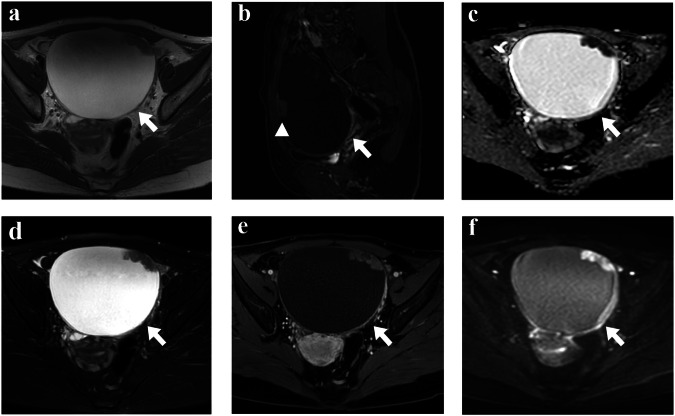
The MR images of a 35-year-old CCC female (FIGO IA stage) with endometriosis were shown as follows. Axial T2WI and axial FS-T2WI (a, d), demonstrated the left ovary had a big unilocular cystic mass with a eccentric nodule (arrowhead), that had a prominent enhancement nodule and little line-like high signal on CE-T1WI with FS (b, e). Axial DWI showed the nodule had a high signal (f), while it had a low signal at ADC map (c)
